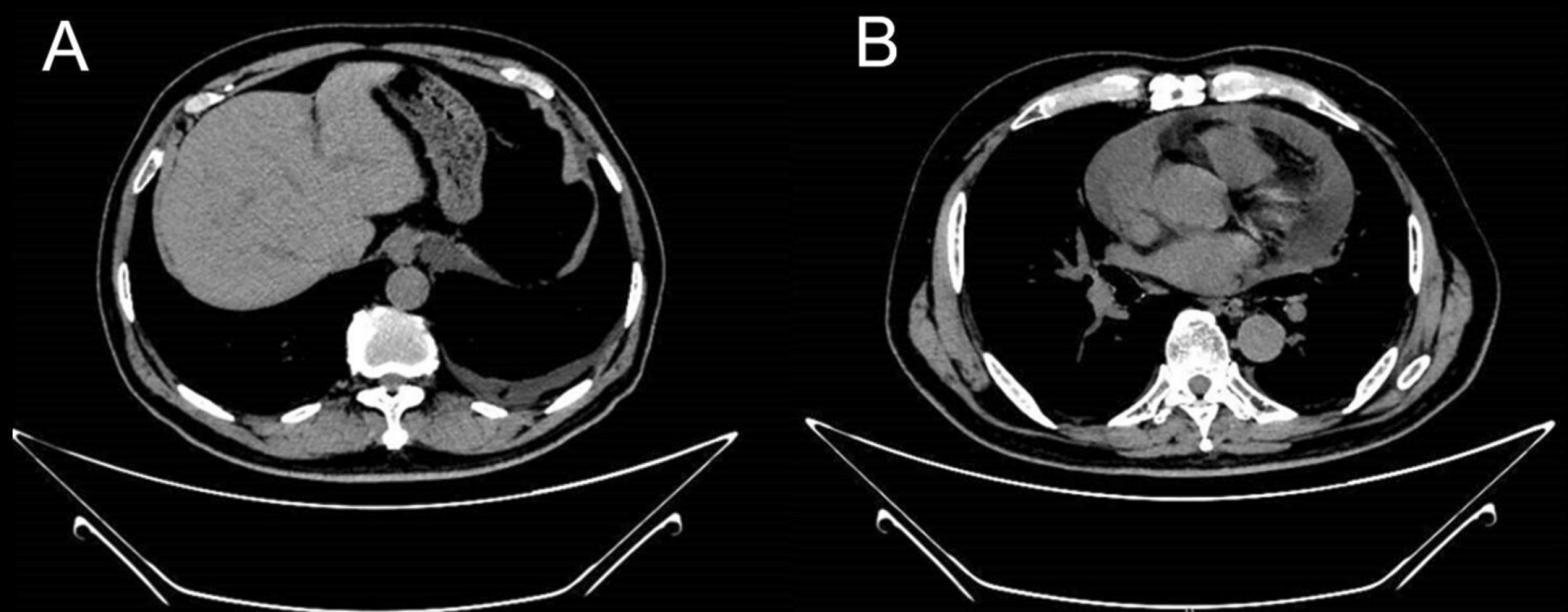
(A,B) Observation of the thorax by computed tomography (CT) showed bilateral pleural effusion and pericardial effusion.
